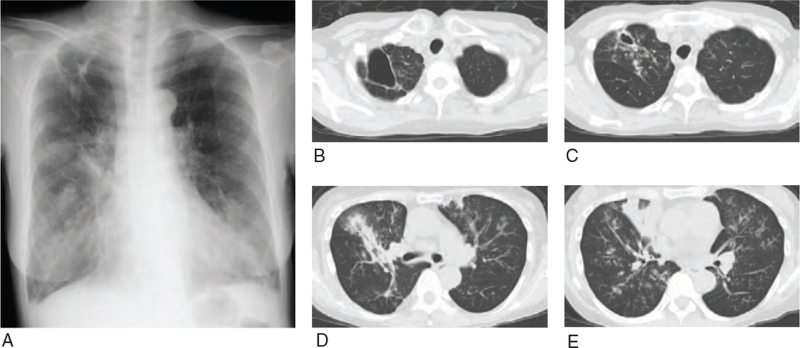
Chest X-ray (A) and chest computed tomography (CT) findings (B–E) on admission. A: Chest X-ray shows a cavity in the right upper lung field and consolidation in the bilateral lower lung fields. B–E: CT scanning shows a cavity in the upper right lobe, consolidation in the middle lobe, and patchy opacities in both lower lobes.
